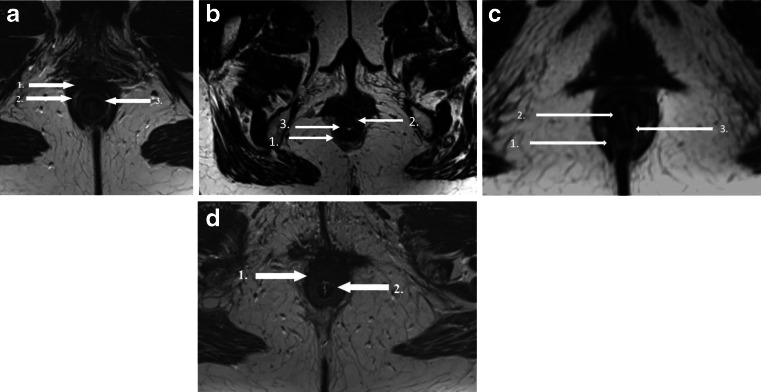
a Axial T2-weighted turbo spin-echo MRI showing a normal external and internal sphincter complex in a 42-year-old woman.* 1* external anal sphincter (EAS);* 2*  conjoint longitudinal coat,* 3* internal anal sphincter. (IAS) b Axial T2-weighted turbo spin-echo MRI showing a defect (defined as a discontinuity of the muscle ring [either isolated or combined, anatomical defect] and/or a hypointense deformation of the muscle ring owing to the replacement of muscle cells by fibrous tissue [functional defect, scar tissue]) of the EAS in a 67-year-old woman. There is also some EAS atrophy.* 1* EAS;* 2*  EAS defect;* 3*  IAS. c Axial T2-weighted turbo spin-echo MRI showing a combined IAS and EAS defect defined as a discontinuity of the muscle ring (either isolated or combined, anatomical defect) and/or a hypointense deformation of the muscle ring owing to the replacement of muscle cells by fibrous tissue (functional defect, scar tissue) in a 31-year-old woman.* 1* EAS;* 2* combined IAS and EAS defect;* 3* internal anal sphincter. d Axial T2-weighted turbo spin-echo MRI showing severe atrophy of the external anal sphincter muscle (≥ 50 % thinning or replacement of the sphincter muscle by fat) in a 67-year-old woman. There is some IAS hypertrophy.* 1*  EAS;* 2*  IAS
